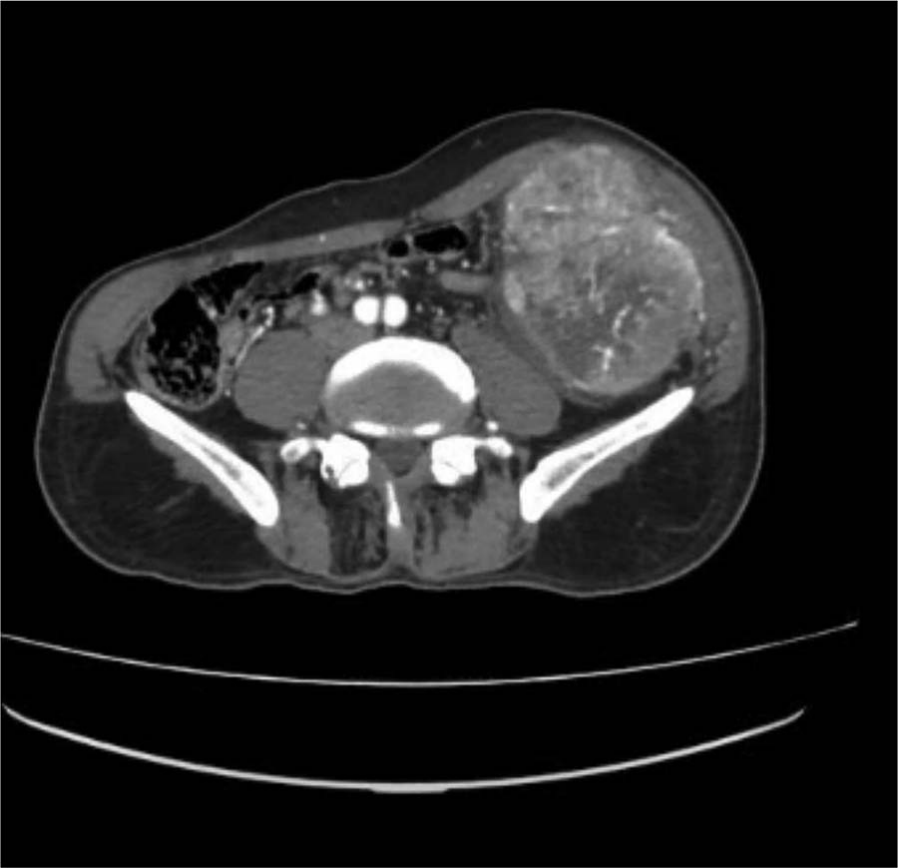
Contrast-enhanced CT scan of the abdomen showing a heterogeneously enhancing soft tissue lesion in the left iliac fossa.
